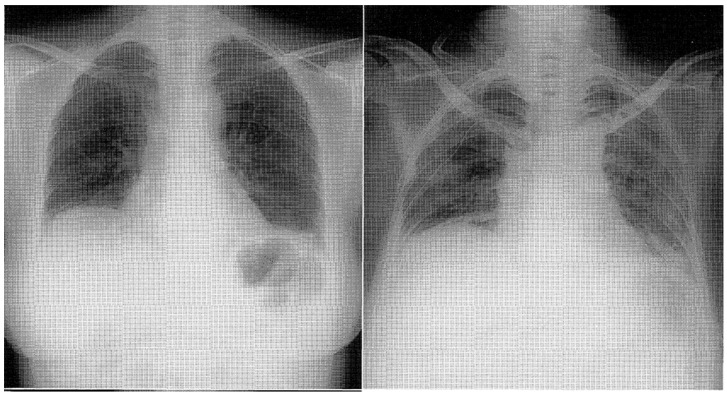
Chest X-ray displaying reticulonodular shadows in both lower lung fields [37].
